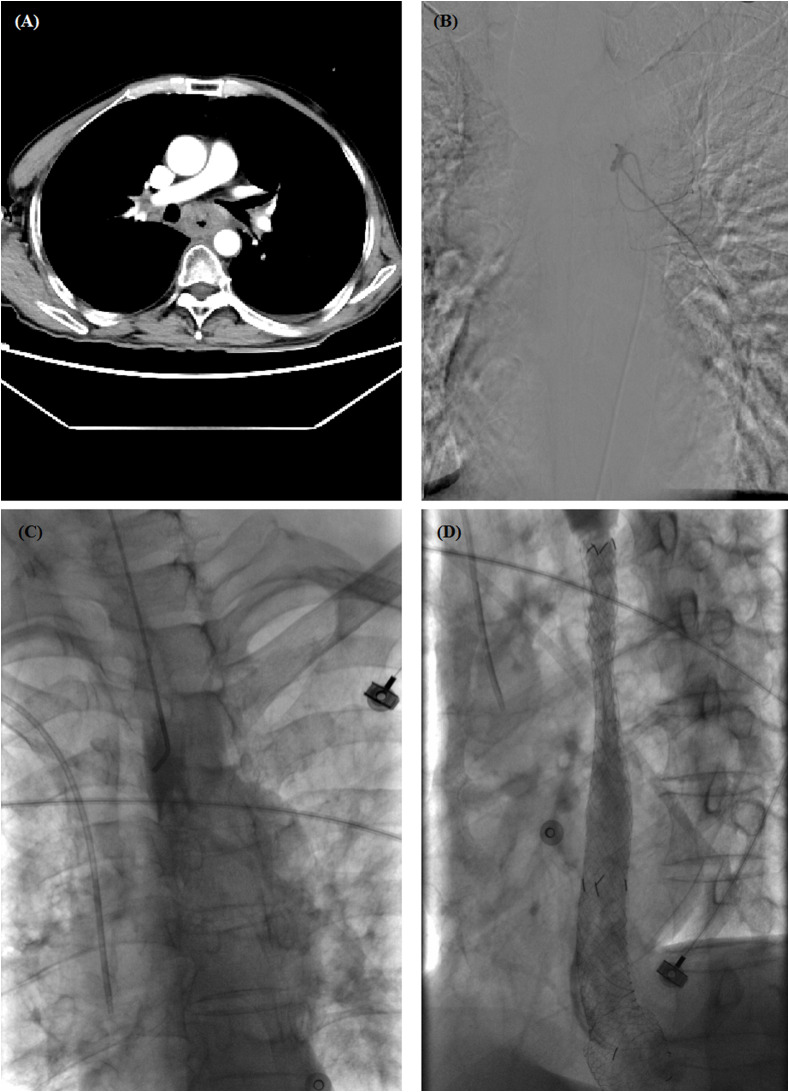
A 60-year-old male with esophageal hemorrhage treated by self-expandable metallic stent (SEMS) insertion. (A) Chest computed tomography (CT) scan showed a thickened wall in the middle esophagus. (B) Transarterial embolization was performed, but hemorrhage did not stop. (C) Esophagography showed complete obstruction in the middle esophagus. (D) Esophagography shows a good position and patency of stent after placement. CT, computed tomography; SEMS, self-expandable metallic stent.
